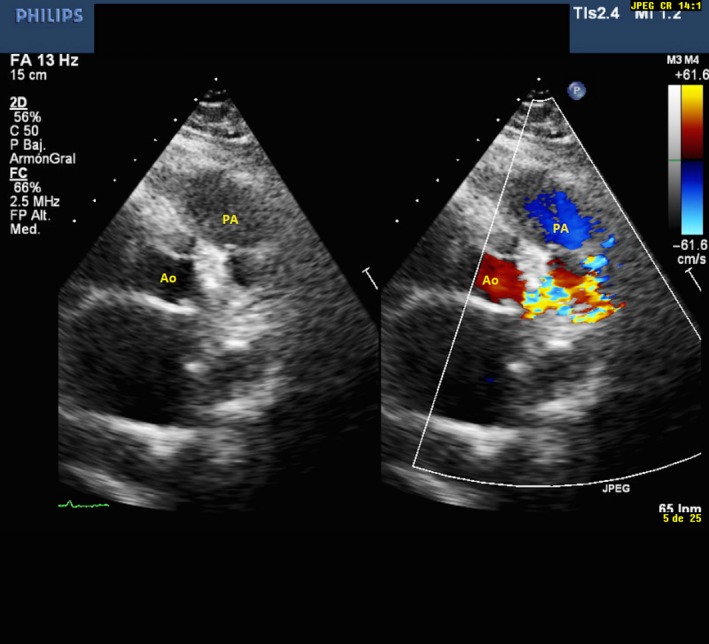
A parasternal short‐axis view of transthoracic echocardiography at the level of the aortic valve (left), showing a turbulent systodiastolic flow, located between pulmonary artery (PA) and aorta (Ao) (right)
